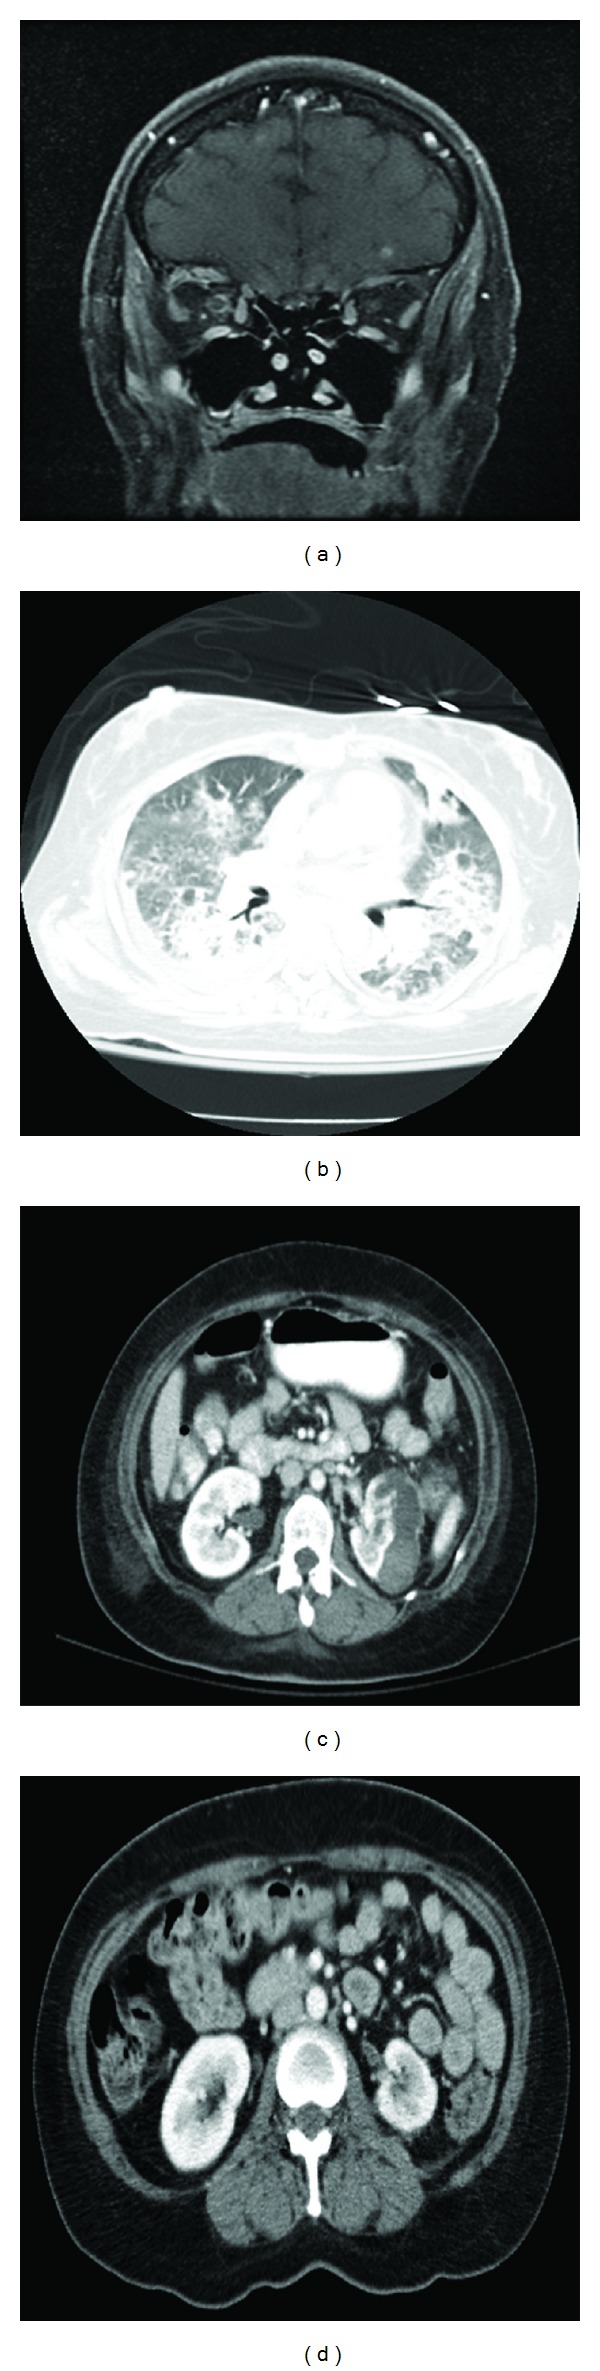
(a) Multifocal contrast enhancing lesions consistent with septic embolic, inflammatory changes surrounding the right ocular globe and enhancement surrounding the right optic nerve. (b) CT chest with IV contrast showing bilateral parenchymal consolidative changes with cavitary lesions. (c) CT abdomen/pelvis with IV contrast depicting left renal abscess, with the largest component in a subcapsular location. (d) Minimal amount of residual fluid adjacent to the left kidney after drainage and systemic antibiotic therapy.
